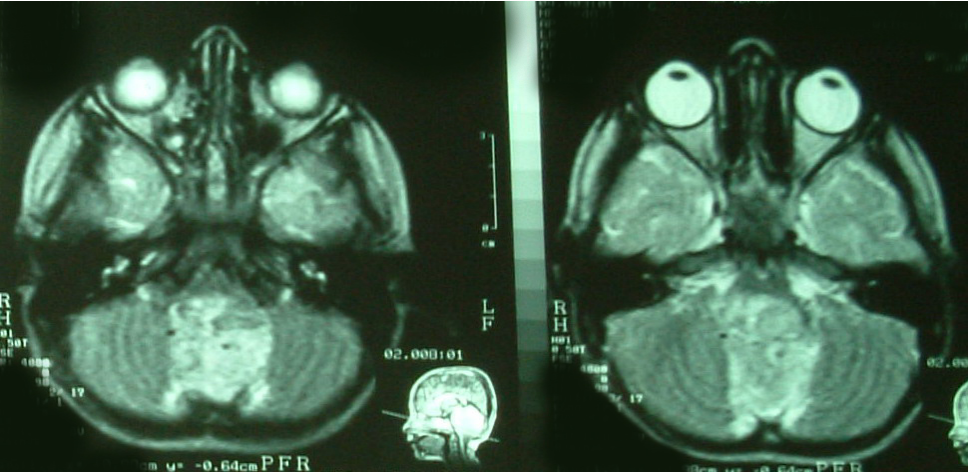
Brain magnetic resonance imaging carried out before the last admission reveals hyperintense mass in T2-weighted image filling the fourth ventricle without any evidence of hemorrhage.
